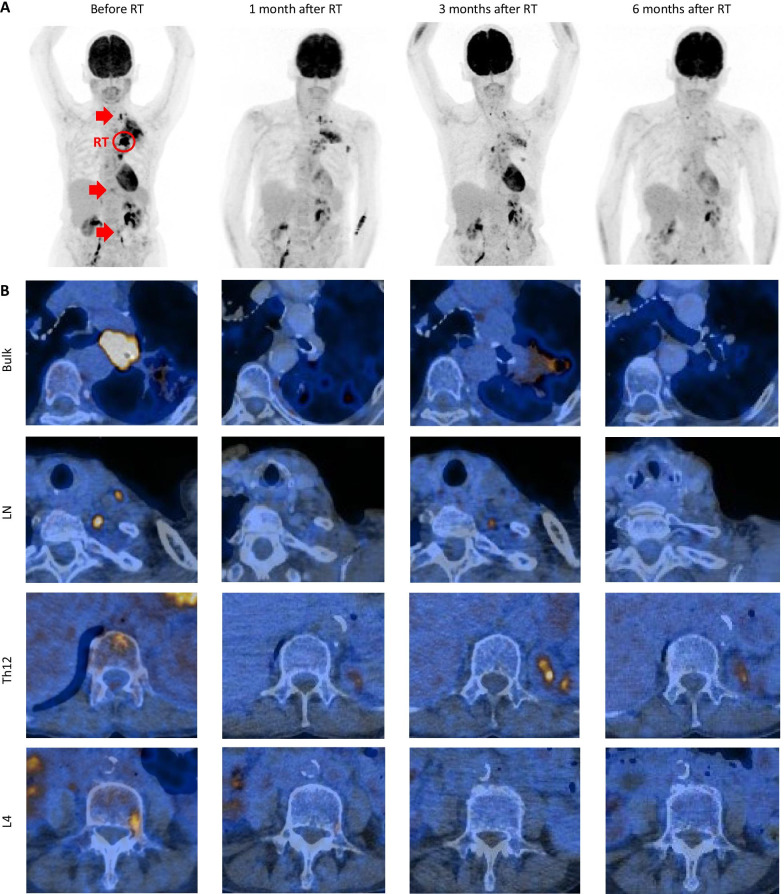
^18^F-FDG PET/CT images before irradiation and at 1, 3, and 6 months after completion of palliative radiotherapy (RT). a PET MIP image and b fused axial PET/CT images showing the initial stenotic pulmonary tumor bulk (red circle), the periclavicular lymph node (LN) metastases, the bone metastases in vertebrae Th12 and L4 (red arrows), and their regression over time. Further follow-up at 9, 12, and 19 months after radiotherapy showed persistent remission
